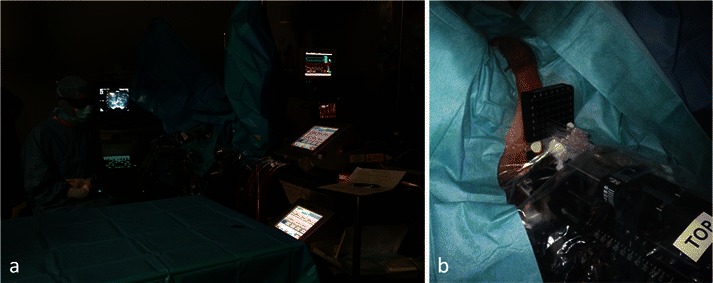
Procedure performed under general anaesthesia, in the darkness (a). Catheters were positioned into the prostate, using TRUS-image guidance in accordance with a previously devised MRI-based treatment plan. Cylindrically diffusing optical fibres were inserted into the catheters (b)
